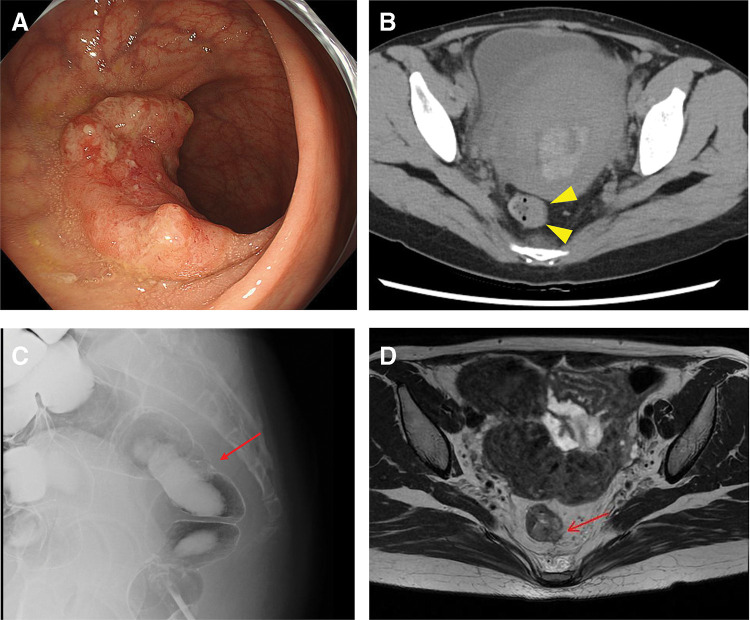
Neoadjuvant chemotherapy and post-delivery findings. (A) Colonoscopy images demonstrating shrinkage of the primary tumor compared to pretreatment findings. (B) Computed tomography confirming reduction in the size of the primary tumor (arrowheads), with no new lesions identified. (C) Barium enema revealing an irregularly elevated lesion with wall deformity in the upper rectum (arrow). (D) Contrast-enhanced magnetic resonance imaging indicating partial rupture and irregularity of the low-intensity muscularis propria (arrow), suggesting a T3 invasion depth.
